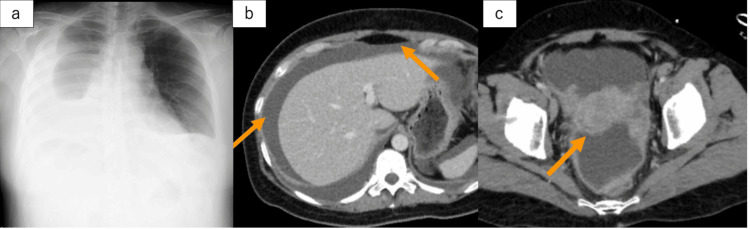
Imaging findings observed at the time of hospital visit (a) Chest radiography showing bilateral pleural effusion with right-sided predominance. (b) Contrast-enhanced CT scan showing ascites and free air in the peritoneal cavity. (c) Contrast-enhanced CT scan showing bilateral adnexal swelling.
